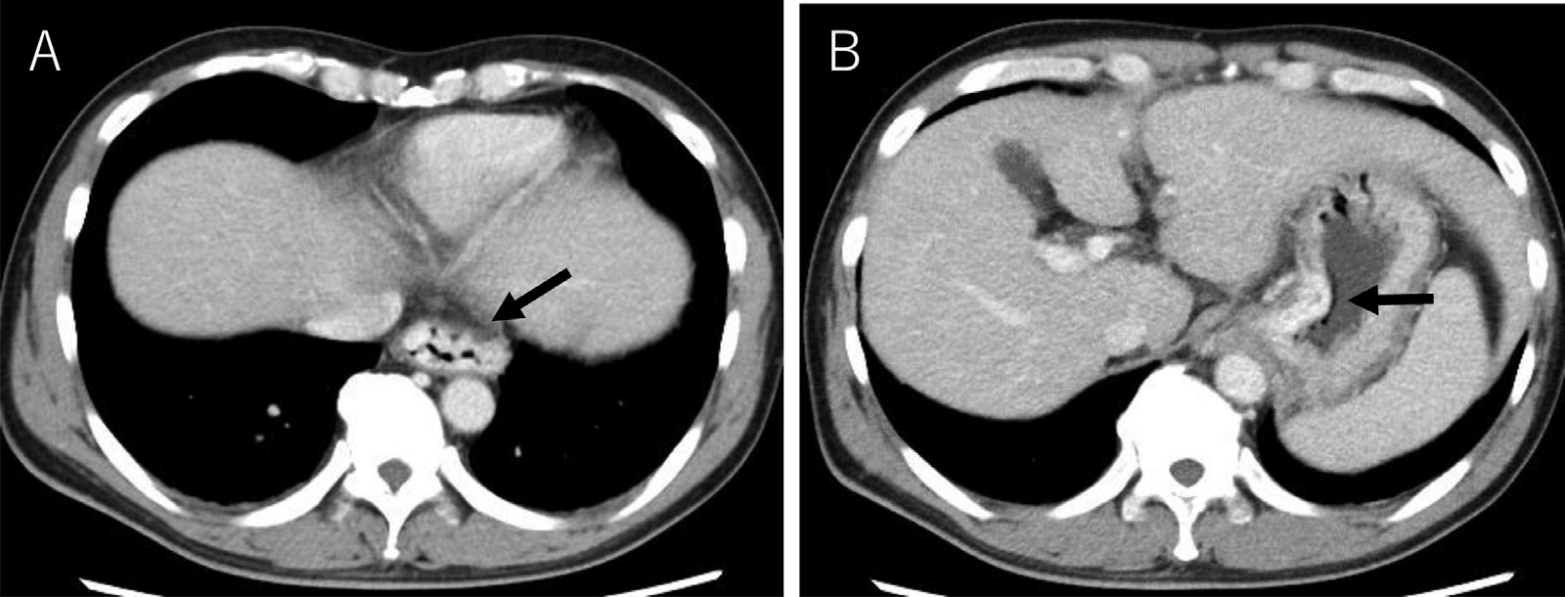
CE-CT image before stepwise PSE and EISML. (A) CE-CT image shows the giant esophageal varices (black arrow). (B) CE-CT image shows gastric varices at the cardia. EISML, endoscopic injection sclerotherapy with multiple ligations; PSE, partial splenic embolization.
